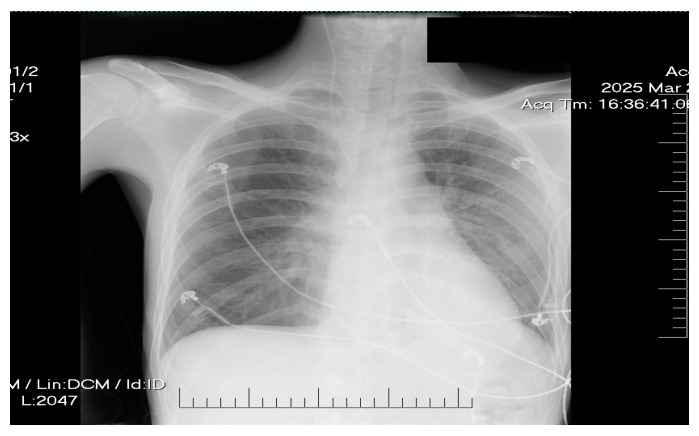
Chest X-ray revealing moderate pulmonary congestion.
